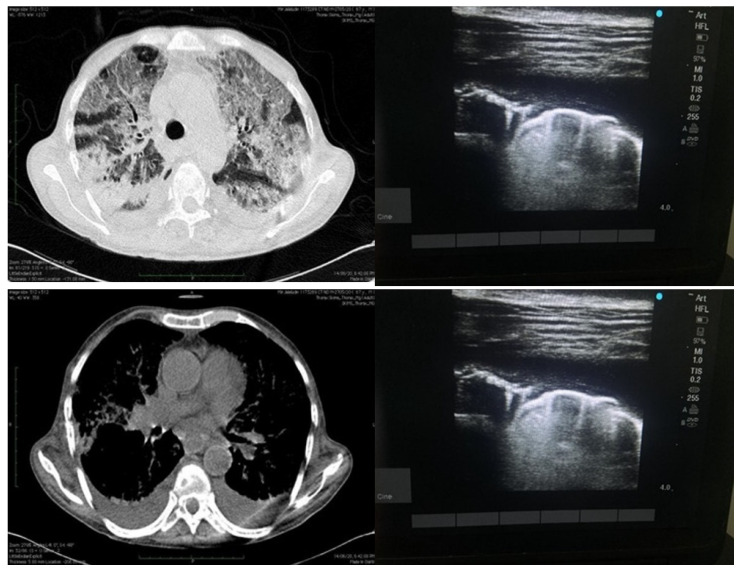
Depicting findings of LUS on right side (above and below) as effusion with irregular pleural line and isolated B-lines and finally small effusion with isolated B-lines as well as confluent B-lines. Image on the left side depicts same patient with HRCT findings of consolidations as well ground glass opacities and pleural effusion.
